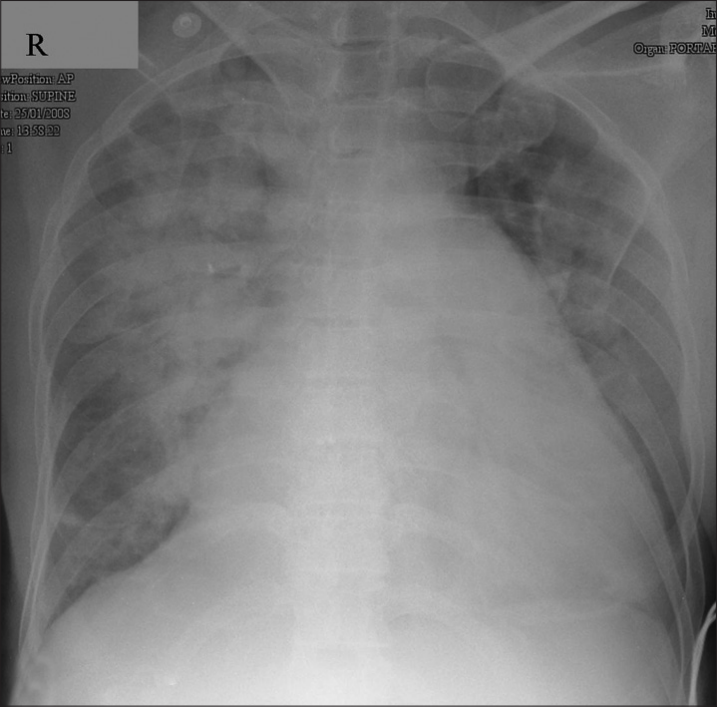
X-ray chest in postero-anterior view 24 hours after atrial septal defect device closure showing established pulmonary edema
